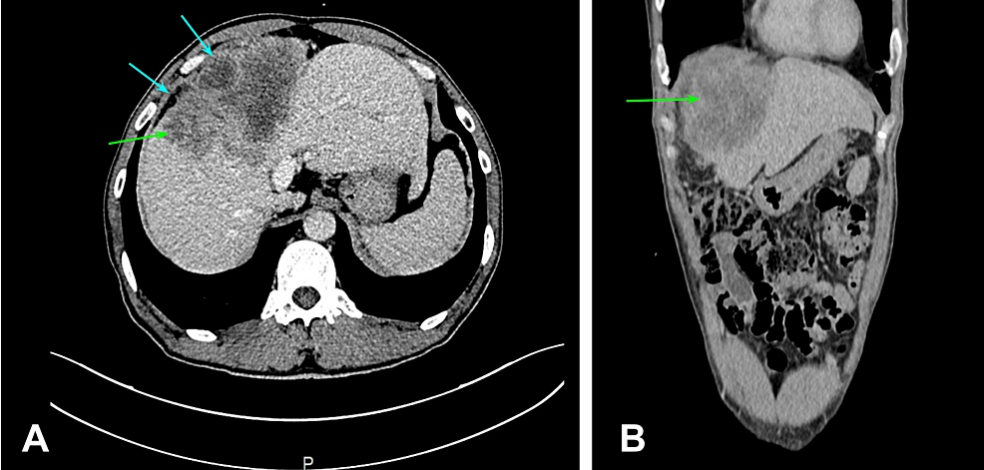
Abdominal CT scan with IV contrast. Liver presented with discretely lobulated contours in some segments, with a heterogeneous appearance at the expense of a neoformation (green arrows) that involves segments IV, VIII and part of segment VII, measuring 13 x 8.6 cm and showing reinforcement after the administration of IV contrast. Images compatible with peritumoral collections were observed, probably derived from tumor necrosis (blue arrows). A: Transverse cut. B: Coronal cut. IV: Intravenous
